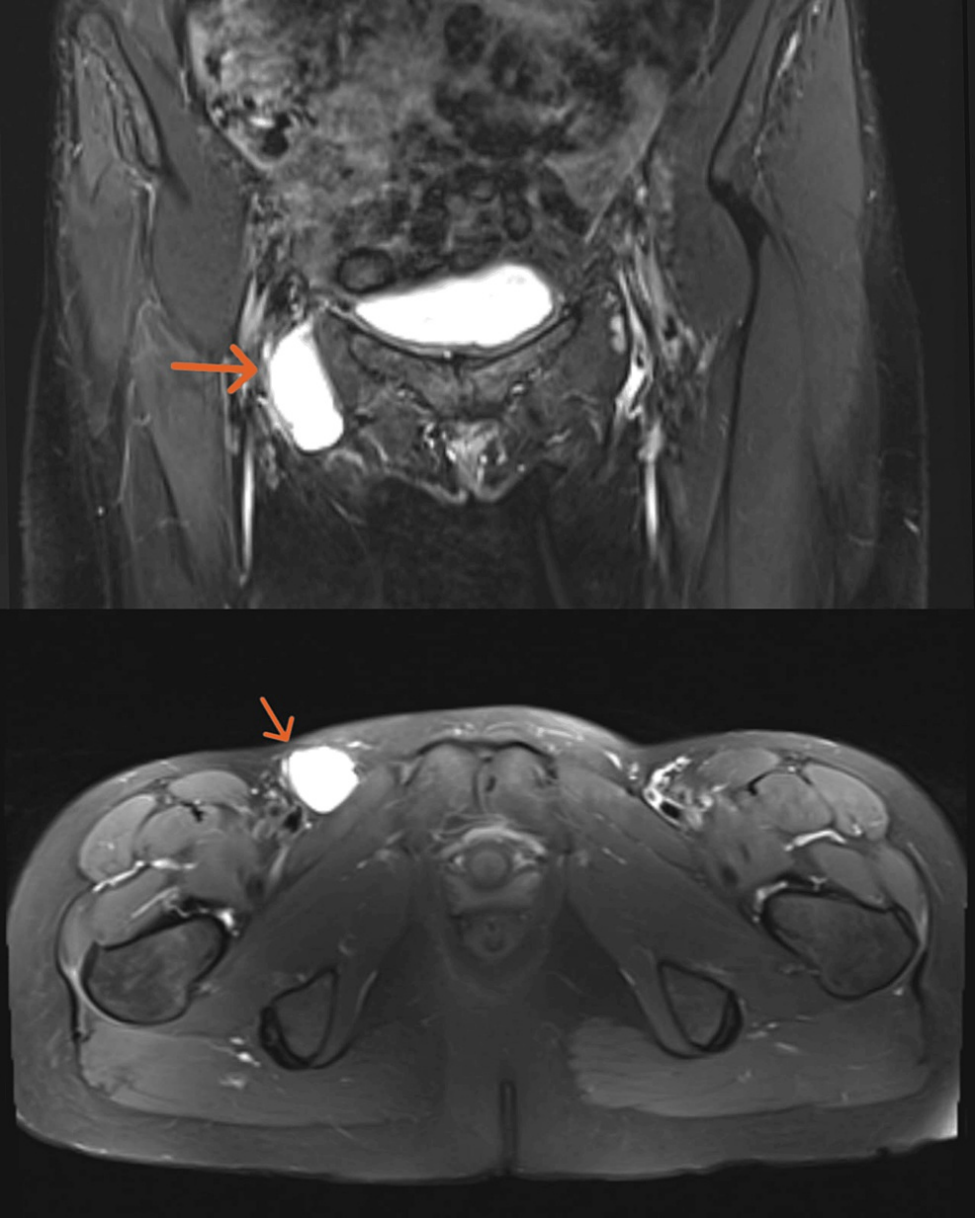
MRI pelvis showing complex cyst at the region of the canal of Nuck (orange arrow) with no evidence of femoral or inguinal hernia
